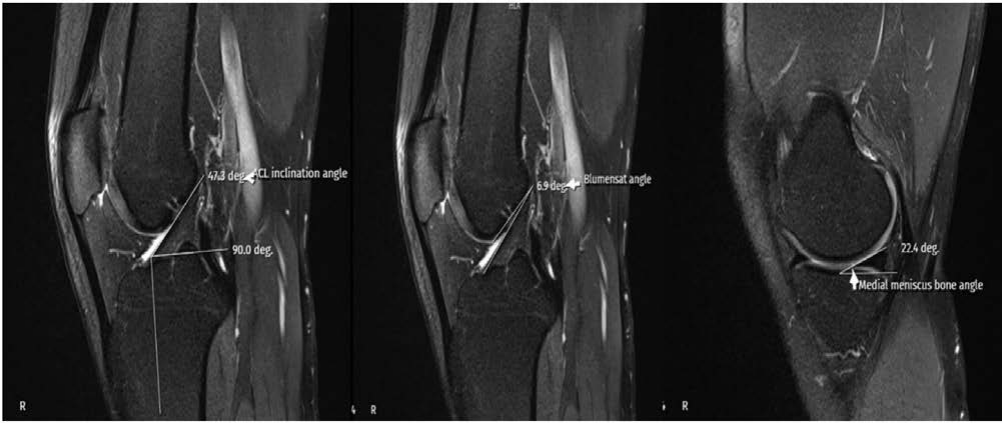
MRI measurement techniques of ACLIA, BA, and MMBA. ACLIA = anterior cruciate ligament inclination angle, BA = Blumensaat angle, MMBA = medial meniscus bone angle, MRI = magnetic resonance imaging.
